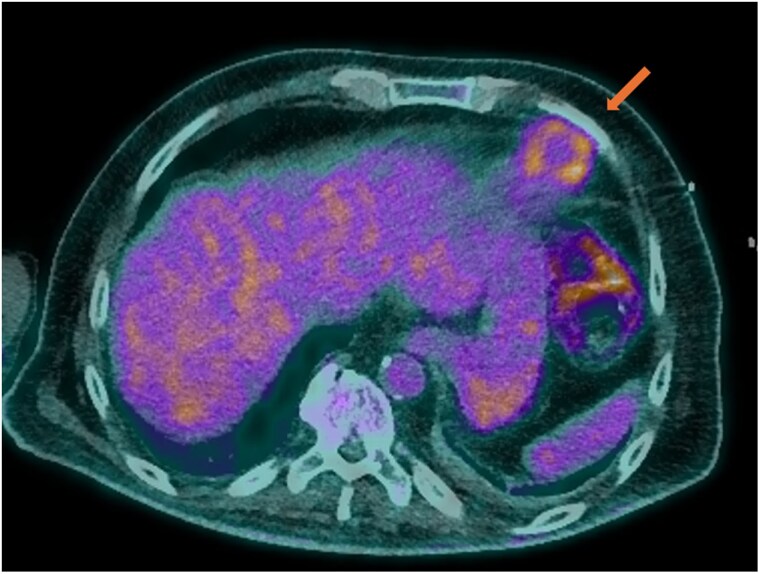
Fused axial PET/CT image demonstrating a circular FDG-avid rim in LV apex.
